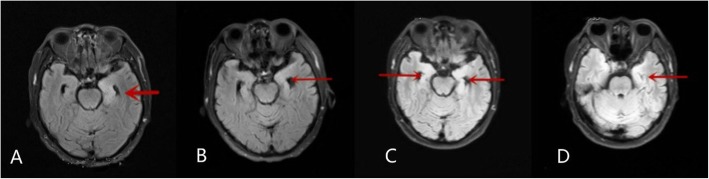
Magnetic resonance imaging of Case nineten. a On admission, magnetic resonance imaging (FLAIR) shows abnormal signals in the left hippocampus, as indicated by the red arrow. b The patient was treated with gamma globulin and methylprednisolone. Following treatment, repeated magnetic resonance imaging shows the signal abnormality was alleviated. c-d Two months later, the patient developed severe memory deterioration. Magnetic resonance diffusion-weighted imaging shows that an enlargement of the lesion to also effect the right hippocampus, as indicated by the additional red arrow
